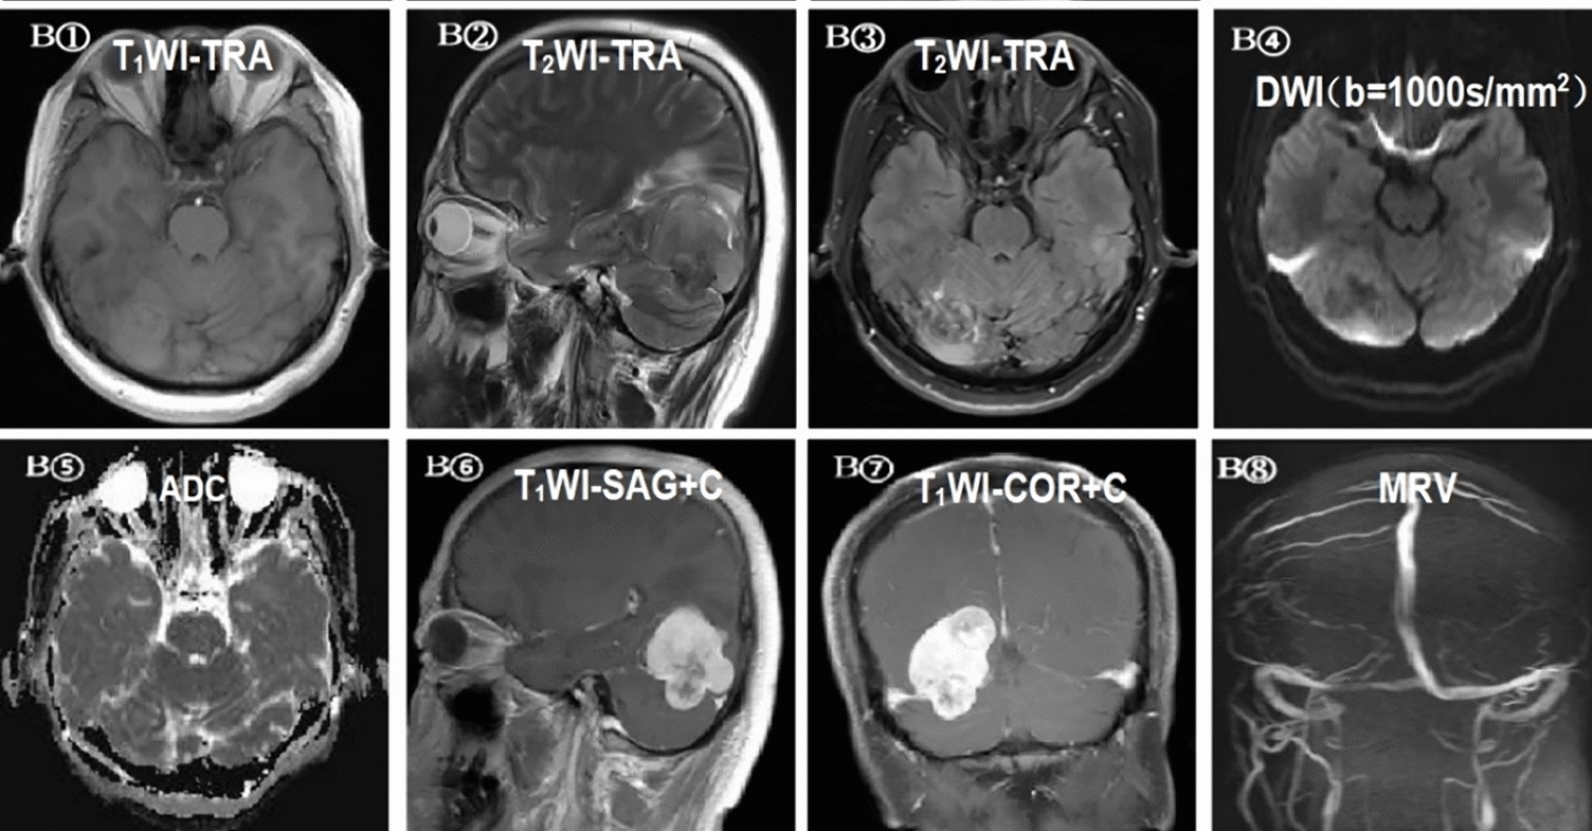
B①–⑧ A 4 3 year-old man with G III ISFT. MRI shows supratentorial and infratentorial solid masses with mixed iso-hypointensity on T1WI and T2WI (B①–②), mixed high- and low-signal intensity on T2-FLAIR (B③), mixed hypointensity on the DWI images (*b* value = 1000 s/mm2) (B④), iso/hyperintensity on the ADC maps (B⑤), and contrast-enhanced MRI (B⑥–⑦) shows obvious inhomogeneous enhancement. (B⑧) MRV images show slight local development in the right transverse sinus. *T1WI, T1* weighted imaging, *T2WI, T2* weighted imaging, *FLAIR* fluid-attenuated inversion recovery, *DWI* diffusion‑weighted imaging, *ADC* apparent diffusion coefficient, *MRV* magnetic resonance venography (*TRA* transverse, *SAG*, sagittal, *COR* coronal)
